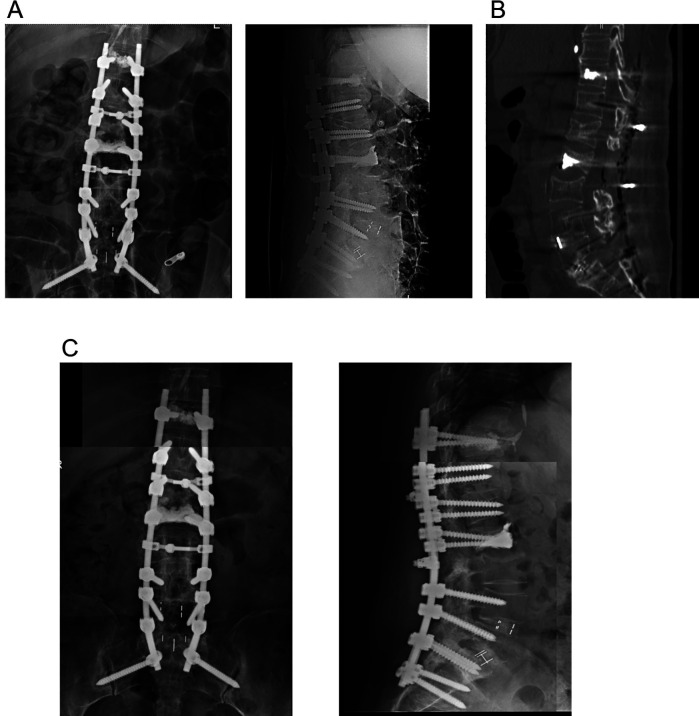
(A) Postoperative posterolateral radiograph after the revision surgery, showing the fixation range from the thoracolumbar vertebrae to the ilium. (B) Postoperative CT scan after the revision operation, showing L2 vertebroplasty, T11 screw tract augmentation, and achieved fusion at L5-S1. (C) Postoperative posterolateral radiograph 6 months postoperatively, taken during scheduled follow-up.
